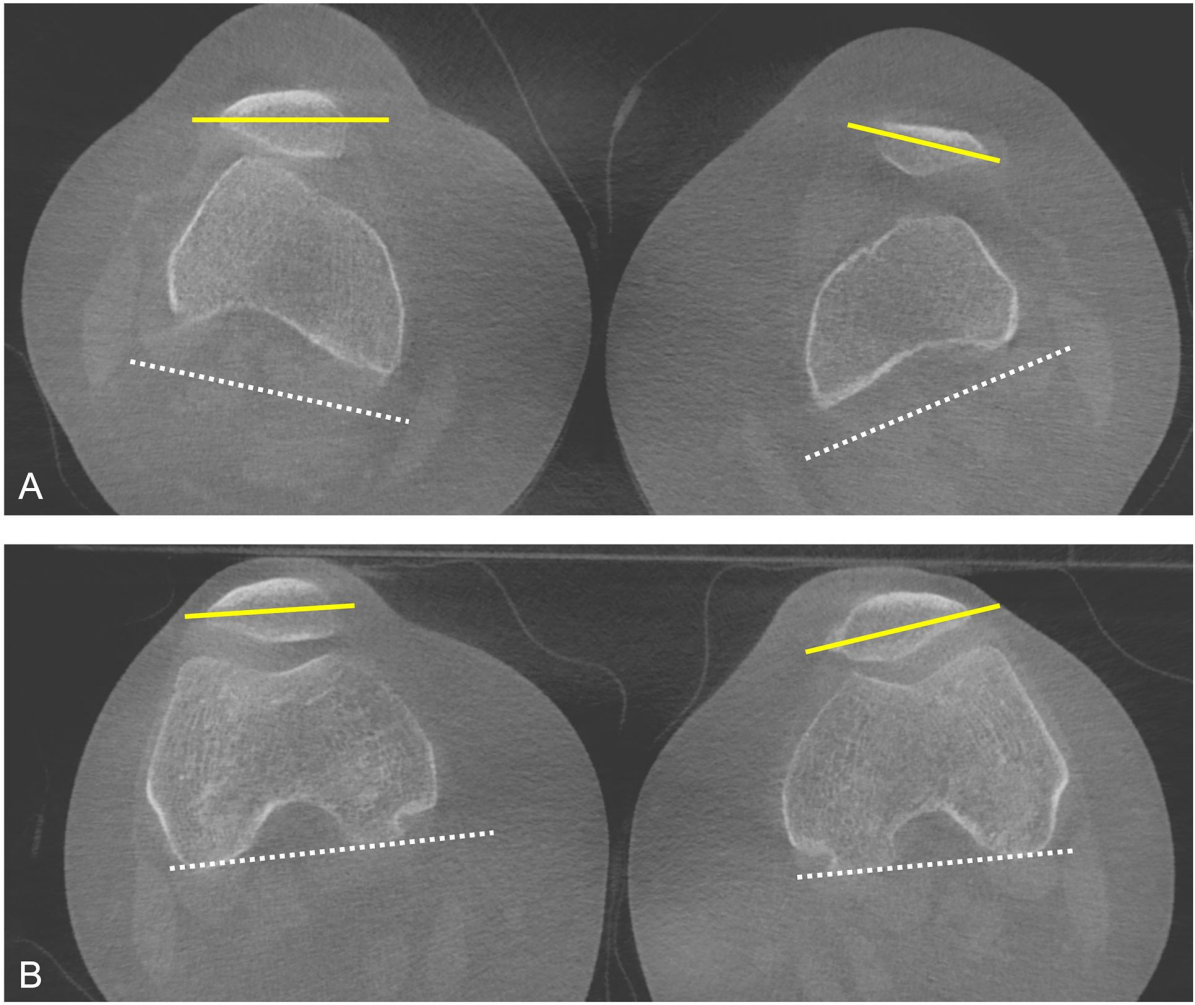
Patient with patellofemoral instability. (A) Weight-bearing CT in full extension demonstrates increased lateral patellar tilt and lateral subluxation. (B) At 30° of knee flexion, WBCT demonstrates patellar reduction with restoration of patellofemoral alignment, reflecting the engagement of the patella within the trochlear groove.
